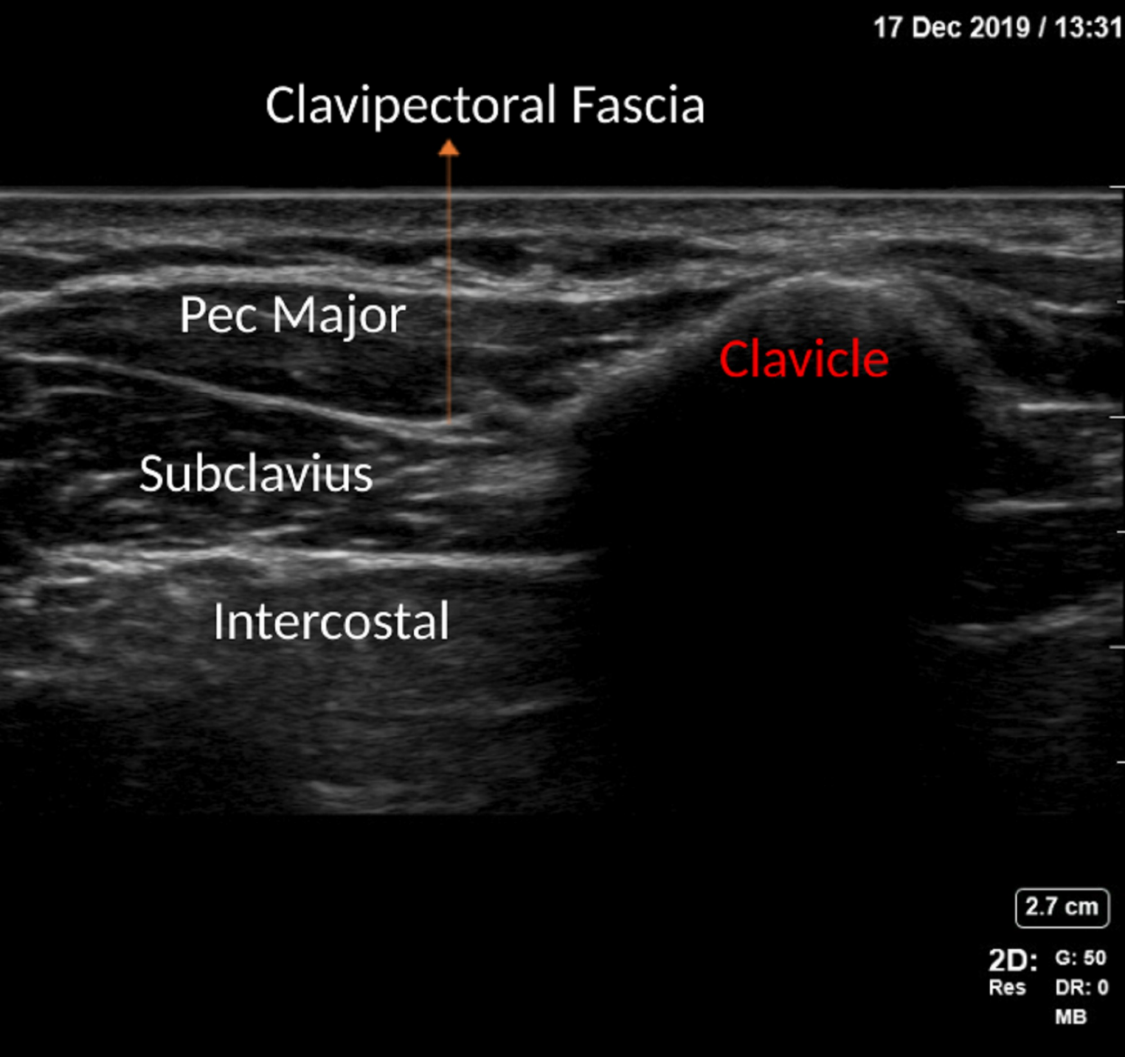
Ultrasound landmarks to identify clavipectoral fascia
